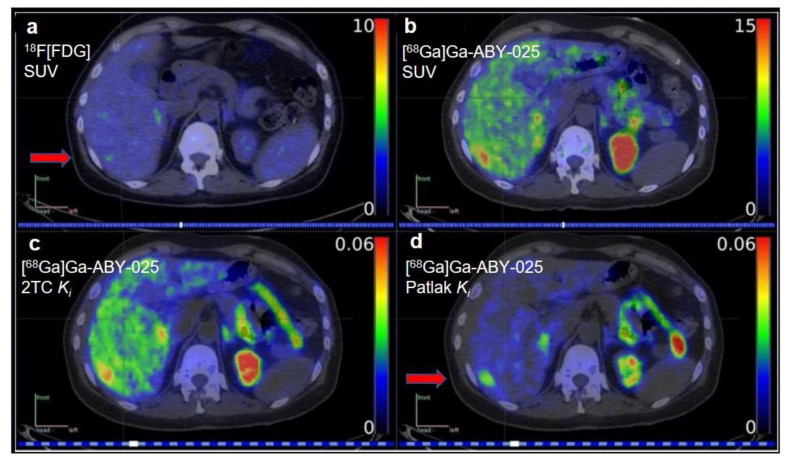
Patlak *K_i_* images of [^68^Ga]Ga-ABY-025 provided good visualization of liver metastases and mitigated nonspecific background uptake in this organ relative to standardized uptake value (SUV) images. (a) [^18^F]FDG-PET, (b) [^68^Ga]Ga-ABY-025-PET, (c) parametric images of 2-tissue compartment (2TC) *K_i_*, and (d) Patlak *K_i_* in a breast cancer patient with multiple small liver metastases in the same patient. (Reprinted from Ref. [18]).
